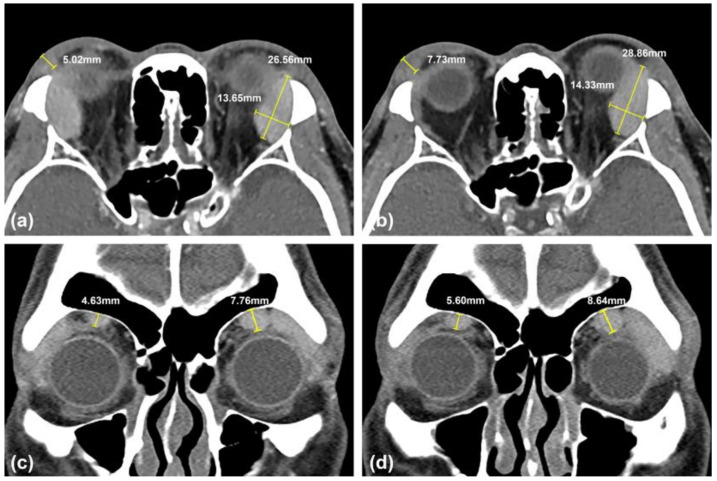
Contrast-enhanced CT of the orbits before orbitotomy (a,c) and six months after orbitotomy (b,d). (a) Axial view revealing bilateral enlargement of the lacrimal gland and right upper eyelid’s homogenous enhancement with an irregular border. The size of the left lacrimal gland was 26.56 mm × 13.65 mm. The thickness of the right upper eyelid mass was 5.02 mm. (b) Axial view revealing >90% reduction of the right lacrimal gland mass. The size of the left lacrimal gland was 28.86 mm × 14.33 mm. The thickness of the right upper eyelid mass was 7.73 mm. (c,d) Coronal view revealing bilateral enlargement of the supraorbital nerve. The diameter of the bilateral supraorbital nerve measured perpendicular to the frontal bone was 4.63 mm for the right eye and 7.76 mm for the left eye (c); 5.60 mm of the right and 8.64 mm of the left (d).
